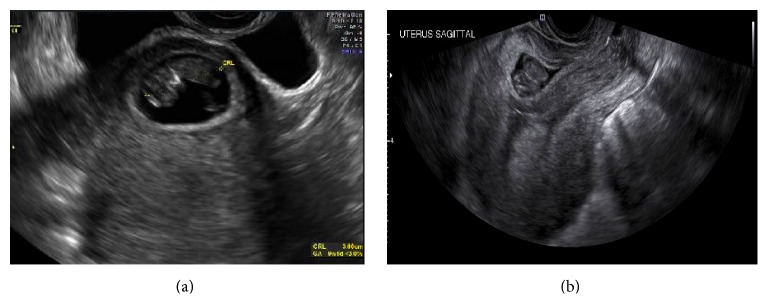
Transvaginal ultrasound showing fetus before treatment (a) and 7 days following uterine artery embolization and systemic methotrexate (b).
